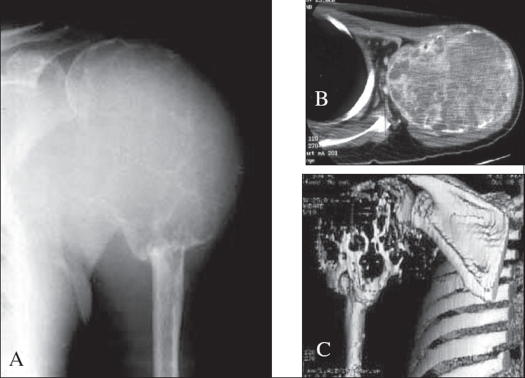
GCT of proximal humerus with extensive cortical expansion and destruction. A) X-rays showing extension to subchondral bone, lysis with trabeculation, and absence of benign host bone sclerosis. B) Axial CT showing multiple areas of cortical erosion. C) 3D reconstruction delineating the spatial extent of the tumour
